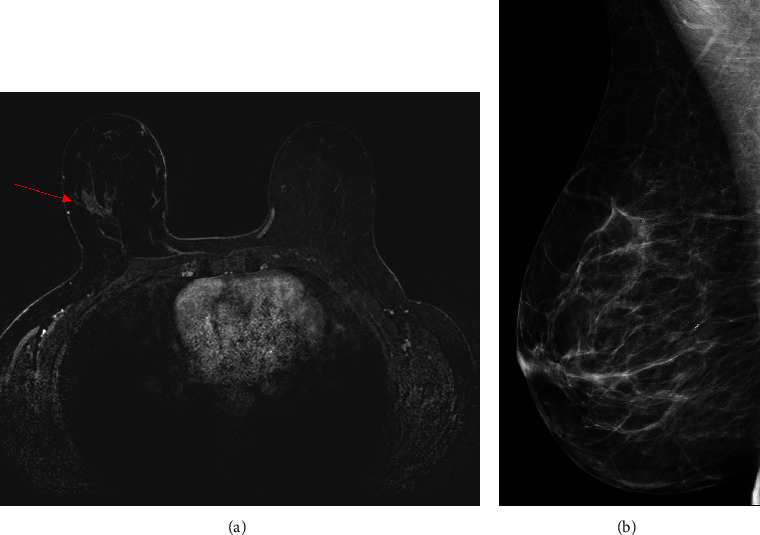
43 year-old BRCA2 carrier, undergoing annual MRI, showing a new NME in the right breast (A axial image, red arrow). MLO view of the right breast shows no corresponding calcifications.
